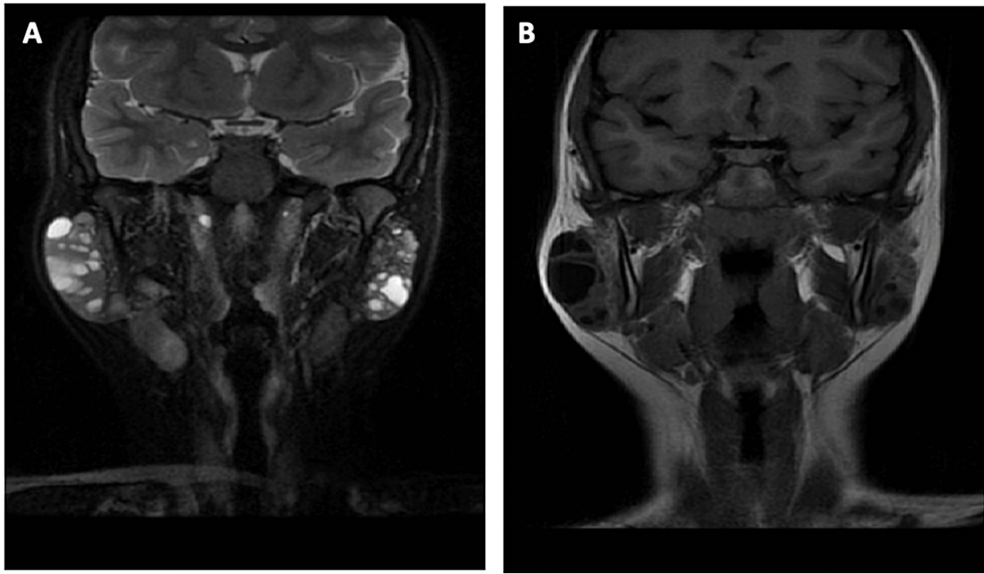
T2-weighted (A) and T1-weighted (B) magnetic resonance imaging head/neck demonstrate numerous cystic lesions in the parotid glands bilaterally Imaging criteria found no pathological lymph nodes.
